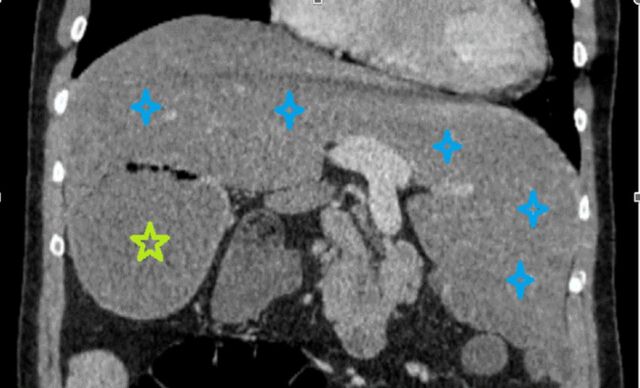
Portal venous phase coronal computed tomography through the hilum of the centrally localized liver and portal vein shows the liver filling the right and predominantly left upper quadrants of the abdomen (right and left liver lobes marked with blue stars). The corpus section of the stomach (green star) is located in the right upper quadrant.
